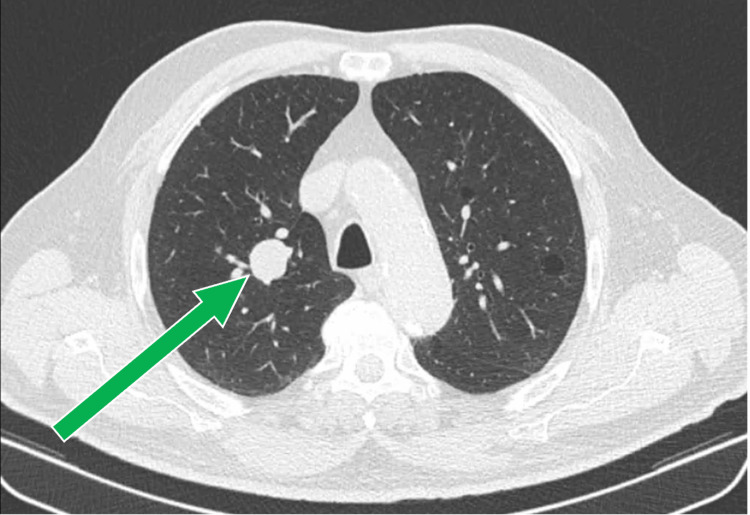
Chest CT - axial plane. The green arrow indicates the right para-hilar lesion.
